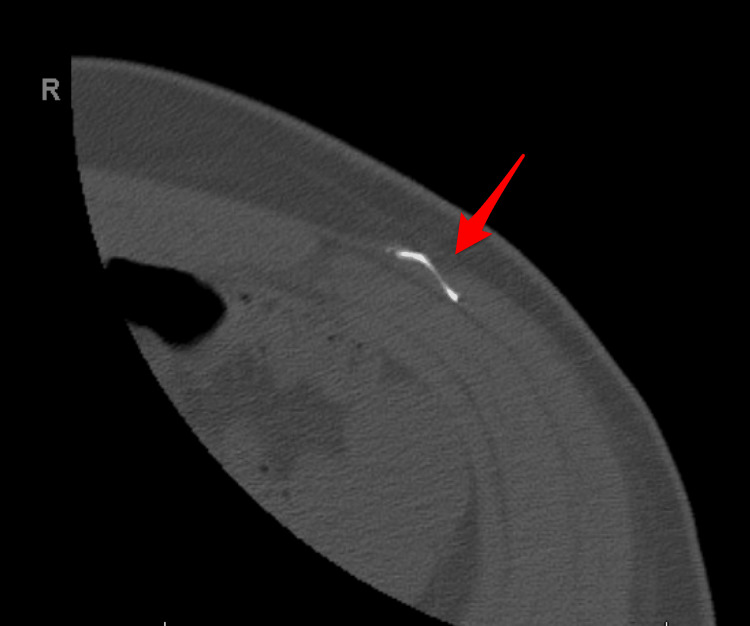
Metal wire in the upper left abdominal wall found on CT scan.
